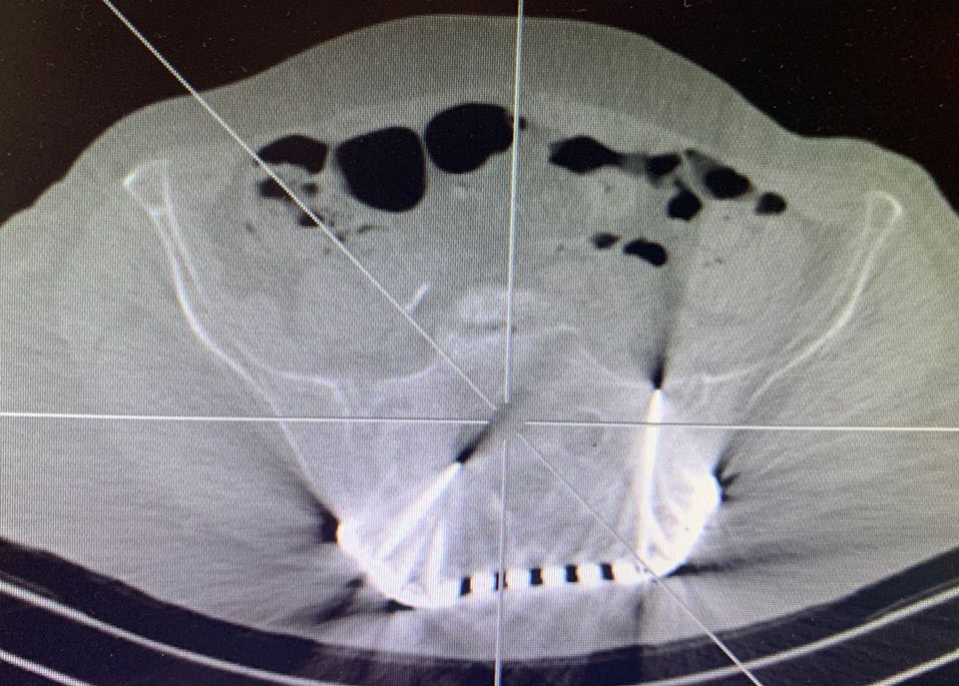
Postoperative CT of the posterior pelvis showing the sacral fractures fixed with ilioiliac plates. One screw on the right side is touching the sacral foramen.
